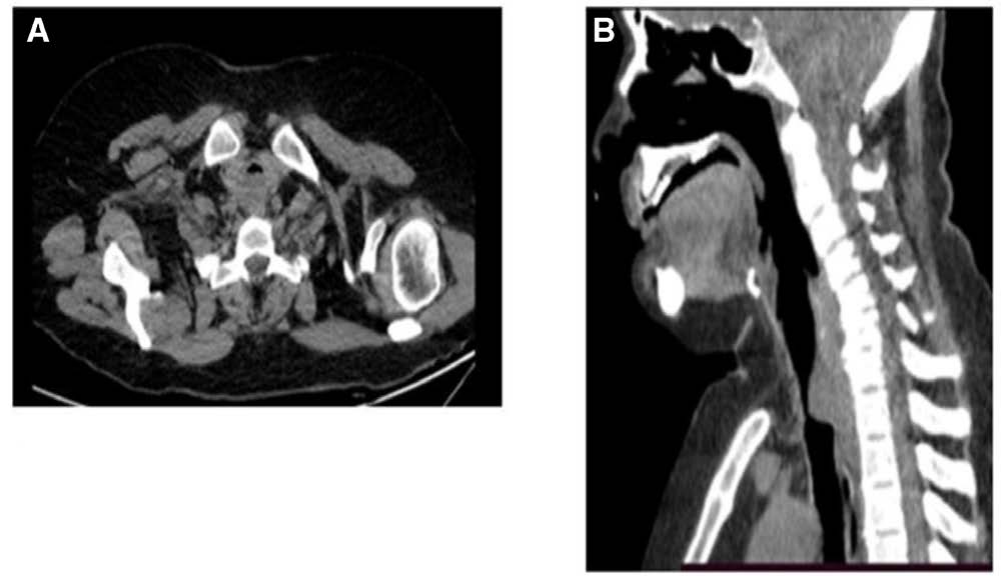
Axial (A) and sagittal (B) non-contrast CT scan images in the mediastinal window showing soft tissue mass narrowing in the tracheal lumen. CT = computed tomography.
